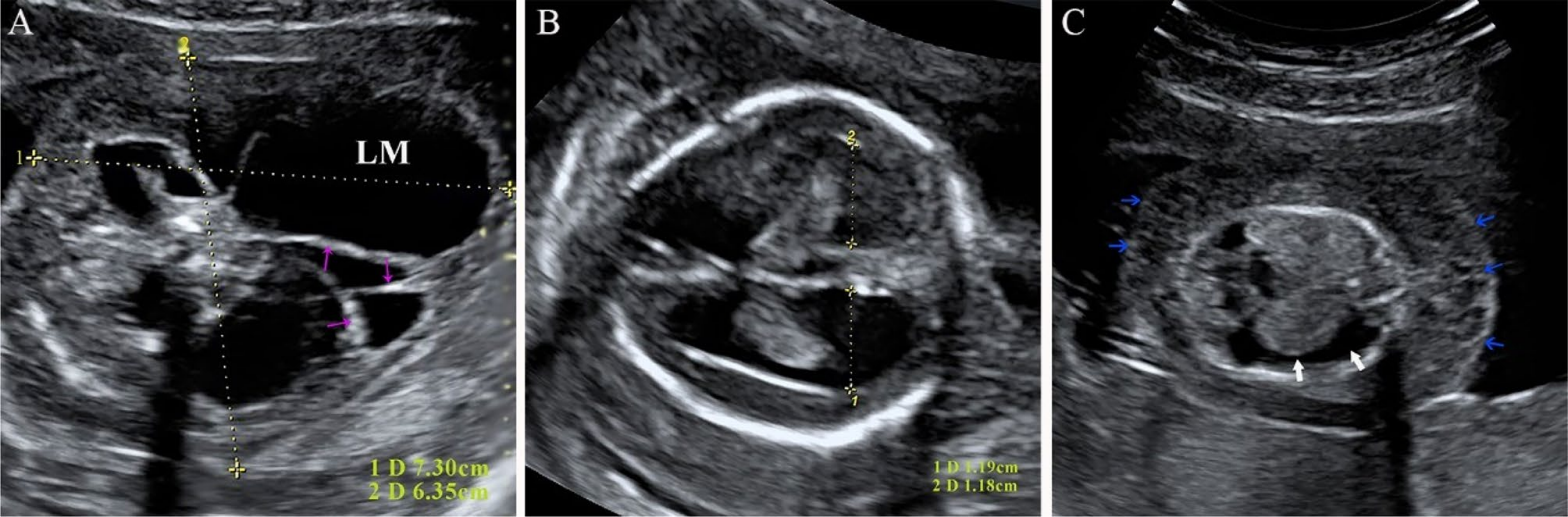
A lymphatic malformation (LM) at 17 weeks of gestational age. (A) Prenatal ultrasound exhibited a large anechoic lesion (7.3 × 6.35 cm) located at the back of the neck, and multiple intervals in the tumor can be identified. (B) The posterior horn of the right and left lateral ventricles were 1.18 cm and 1.19 cm, respectively. (C) Ultrasound of fetal chest showed a large pleural effusion (white arrow) and demonstrated severe fetal subcutaneous edema with low unevenness echo (blue arrow).
